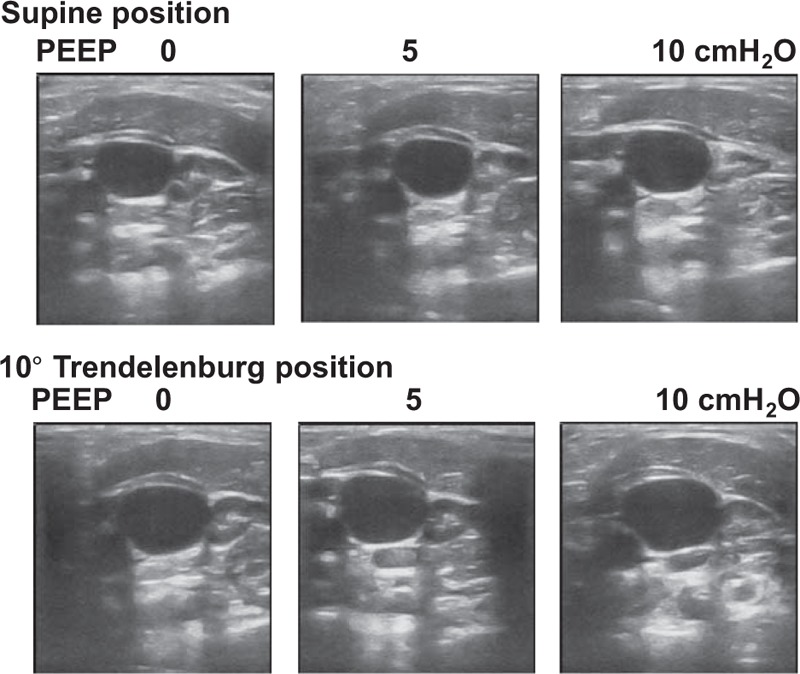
Ultrasound images of the right internal jugular vein.
